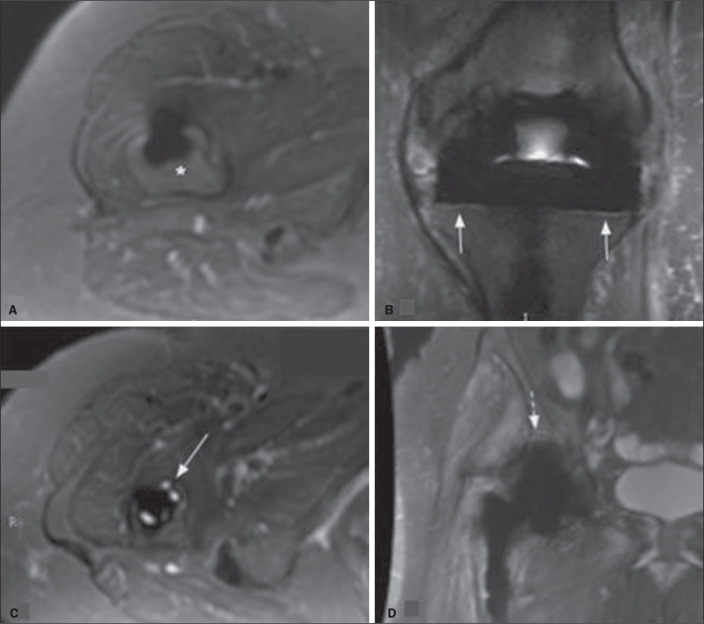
Types of interfaces on MRI (MAVRIC-SL sequences). A: Axial STIR
